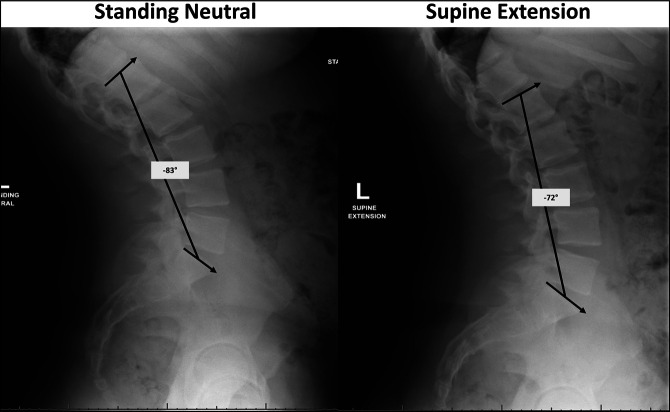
Preoperative lateral lumbar supine extension and standing neutral radiographs demonstrating higher lumbar lordosis when standing versus supine in extension.
